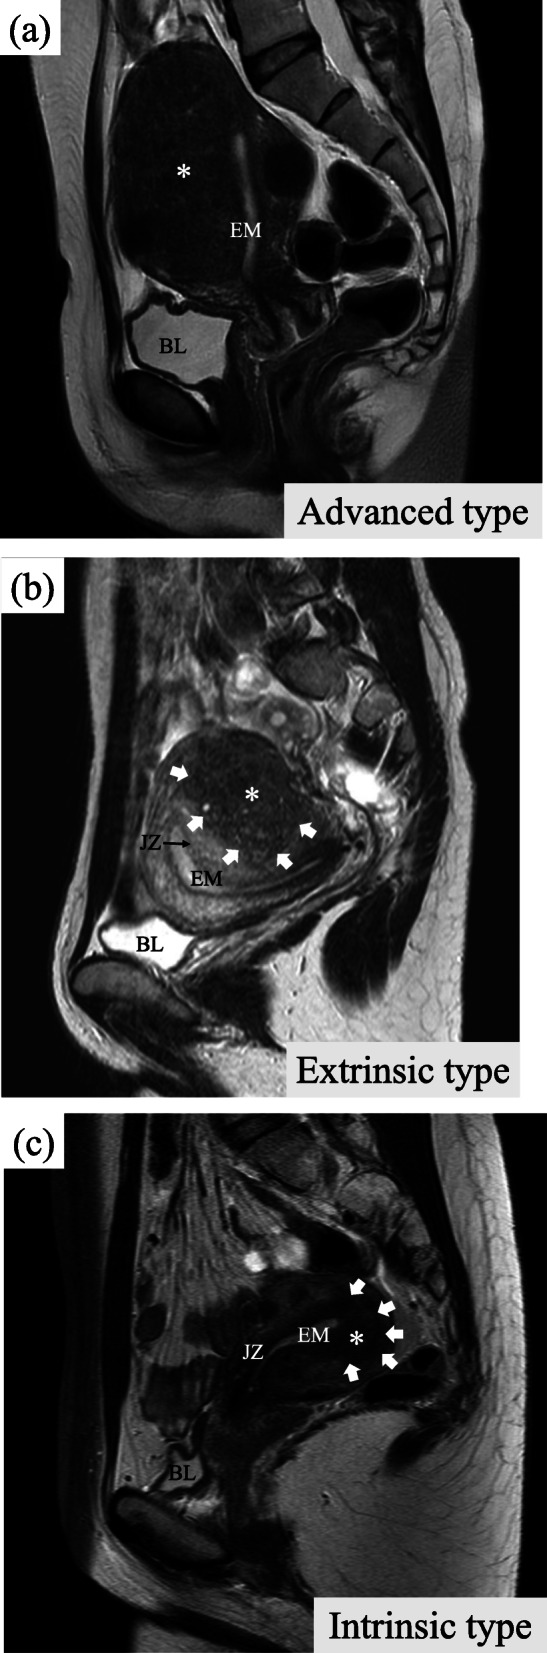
Representative sagittal T2-weighted MRI for each subtype. a Advanced type: the adenomyotic lesion invades the endometrium from the serosa through the full thickness of the myometrium. b Extrinsic type: the adenomyotic lesion is localised to the serosal side of the posterior wall of the uterus. A normal myometrium exists between the adenomyotic lesion and the junctional zone. The arrows show the border between the adenomyotic lesion and the uterine myometrium. c Intrinsic type: the adenomyotic lesion is localised to the endometrial side. The myometrium external to the lesion is intact. The arrows show the border between the adenomyotic lesion and uterine myometrium. MRI, magnetic resonance imaging; EM, endometrium; JZ, junctional zone; BL, bladder. The asterisks show the adenomyotic lesion
